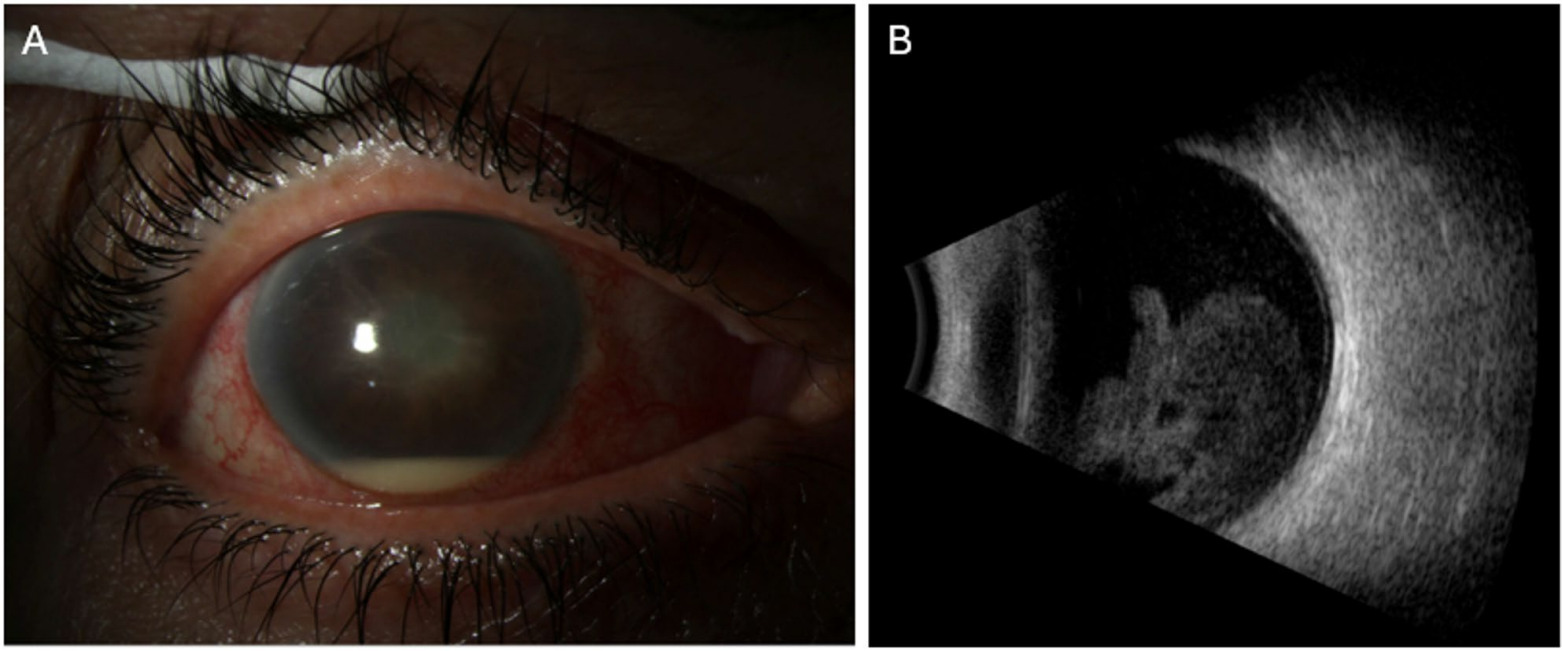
Patient 5: A 71-year-old female presented 4 days after cataract surgery in the right eye. Visual acuity was light perception. (A) Examination demonstrated diffuse conjunctival injection, corneal edema, and a hypopyon. (B) B-scan ultrasound revealed dense mobile vitreous opacities. The patient underwent vitreous tap and injection with vancomycin, ceftazidime and dexamethasone. Best-corrected visual acuity at last follow-up was 20/40.
